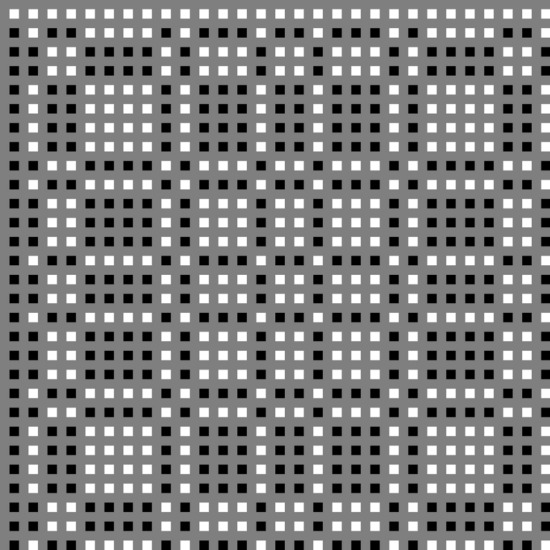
Visual representation of the decoding array used to reconstruct the X-ray spot image. Black is − 1, grey is zero, and white is + 1.
